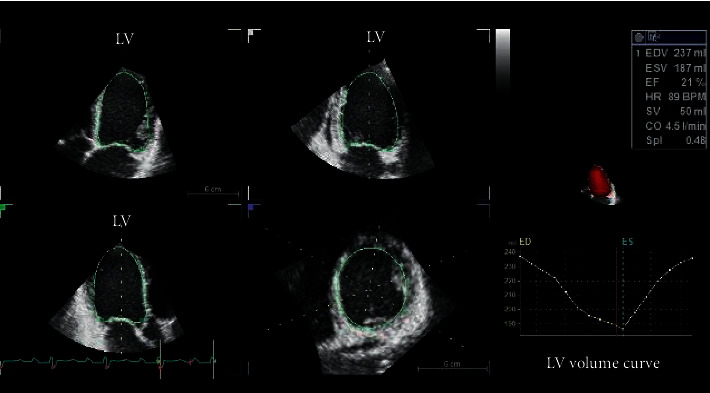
Left ventricular quantitative analysis using 3D transthoracic echocardiography in a patient with dilated cardiomyopathy and functional mitral regurgitation. LV: left ventricle.
